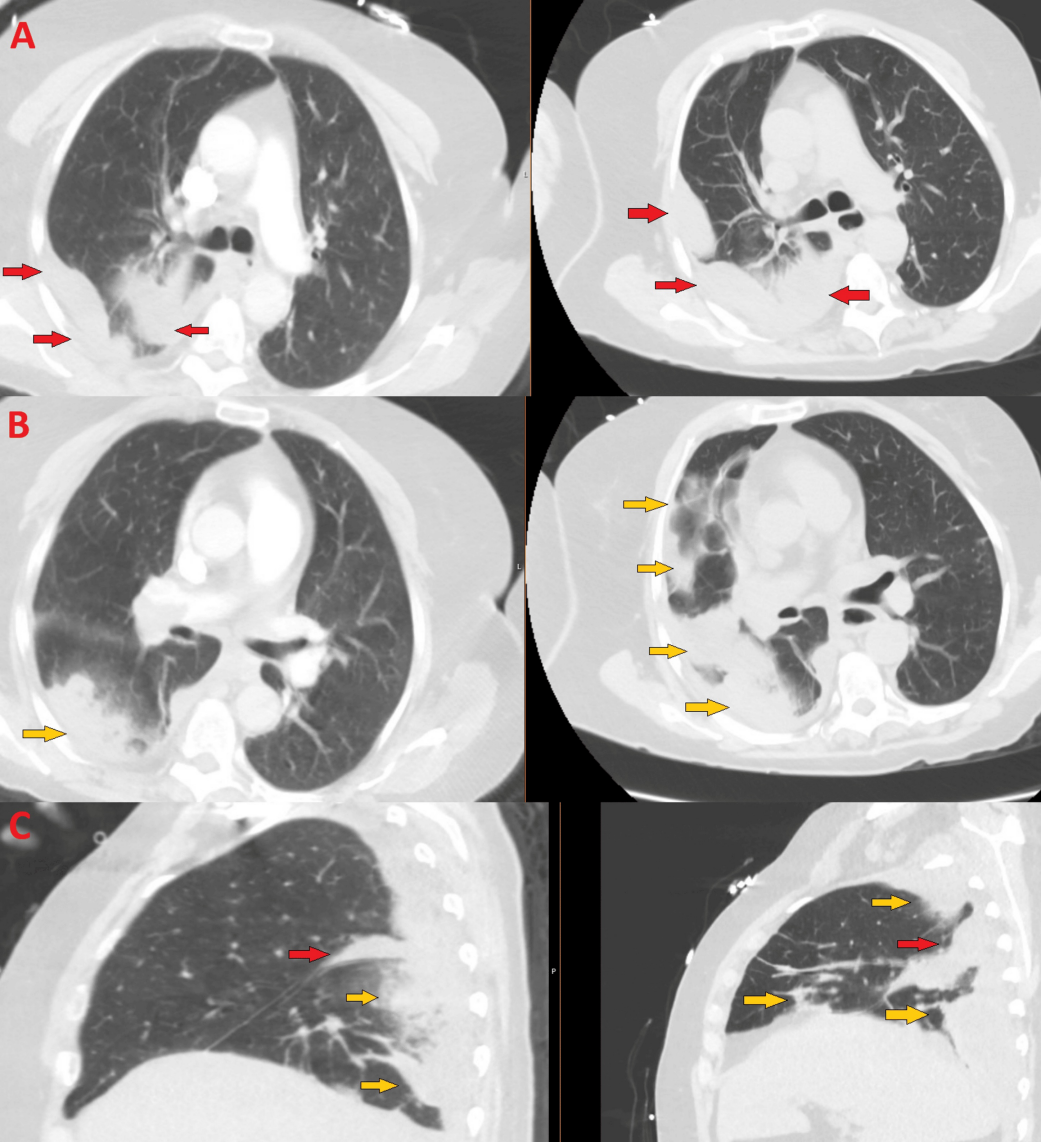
Worsening pulmonary infarct on rapid response Comparison of axial cuts (A, B) and sagittal cut (C) of CTA Chest on initial ED imaging (left) vs rapid response (right) demonstrating worsening pulmonary infarct (yellow arrows), stable/slightly worsening small pleural effusions (red arrows). Formal radiologist interpretation: "Redemonstrated right main pulmonary trunk and right lower lobe segmental pulmonary emboli. No evidence of right heart strain. Evolving right lower lobe pulmonary infarct. Right pleural effusion, increased compared to prior."
